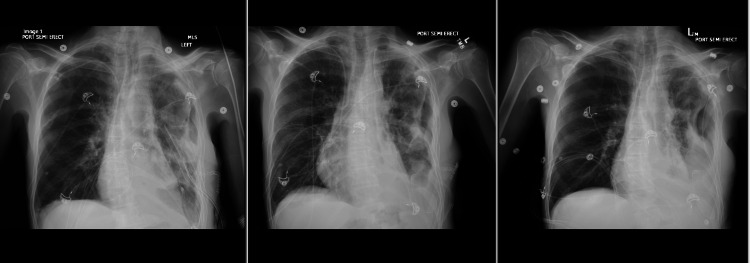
Daily Chest X-rays post video-assisted thoracic surgery (VATS) procedure
